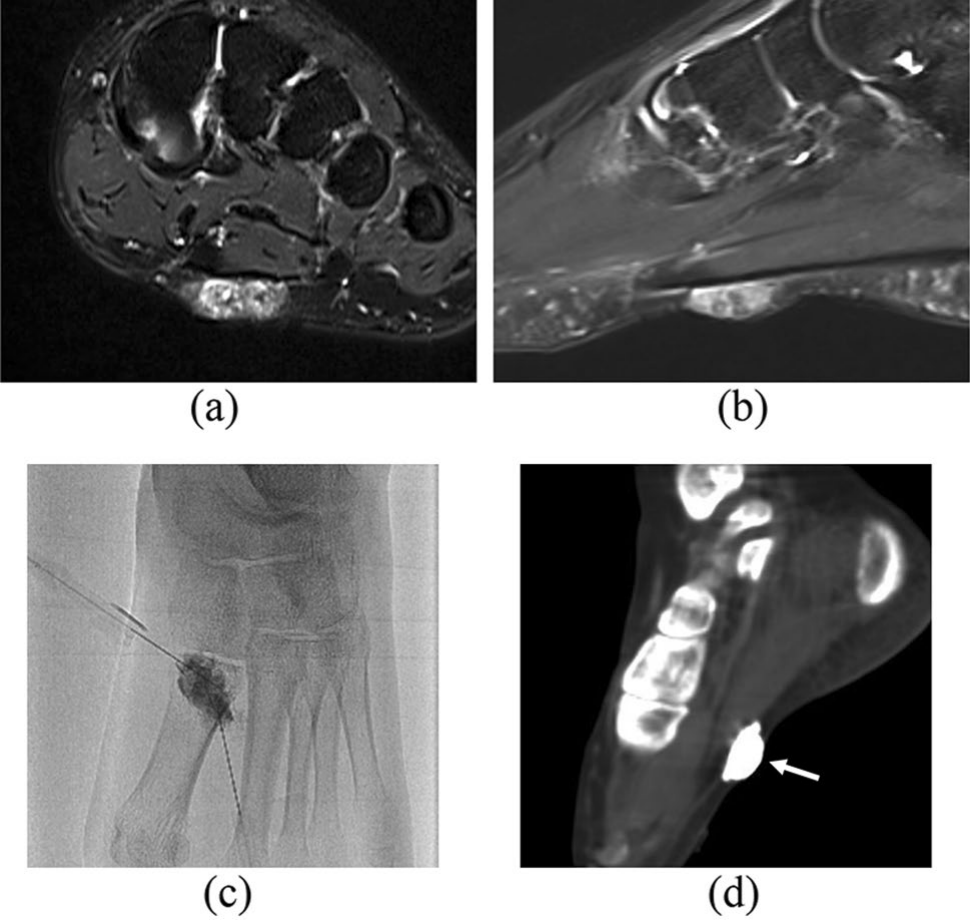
Case of a 28-year-old woman with a VM in her left foot. Axial a and sagittal, b FS-T2WI images show a hyperintense well-defined and cavitary lesion. The lesion measures 18 mm in the longest diameter. A drainage vein is absent on FS-T2WI. c A direct puncture venogram shows no visualization of a drainage vein. d After 3% polidocanol sclerotherapy, a cone beam CT image demonstrates dense pooling of contrast medium without a drainage vein (arrow). The clinical outcome was “free of pain”
